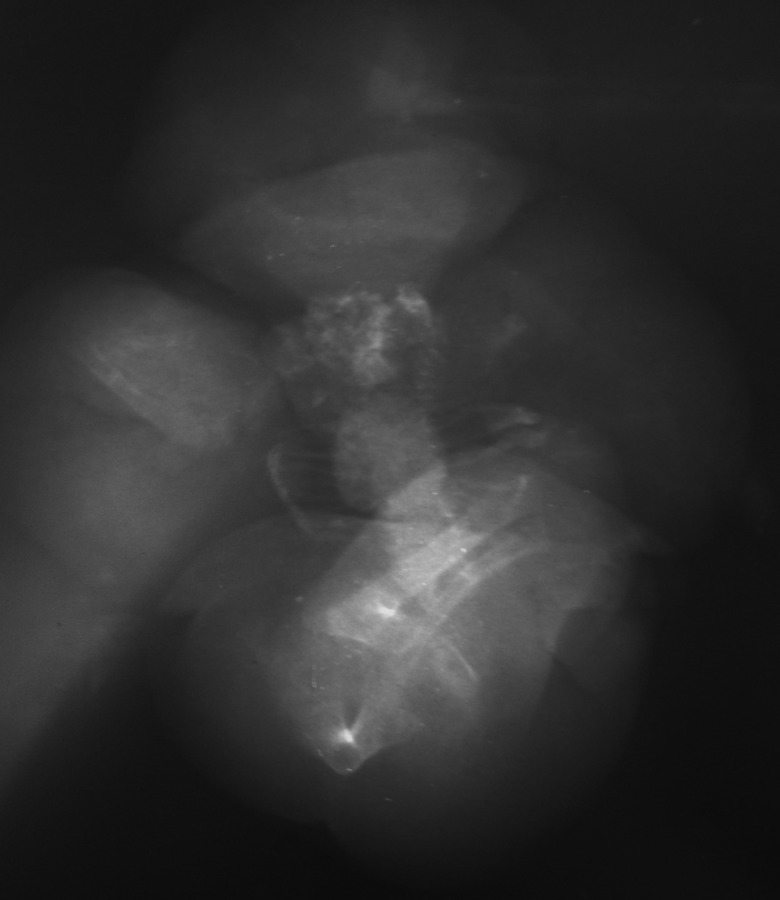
Figure 5: Radiograph of FIF showing ribs, vertebrae, hip bone, bones of face and limbs.
